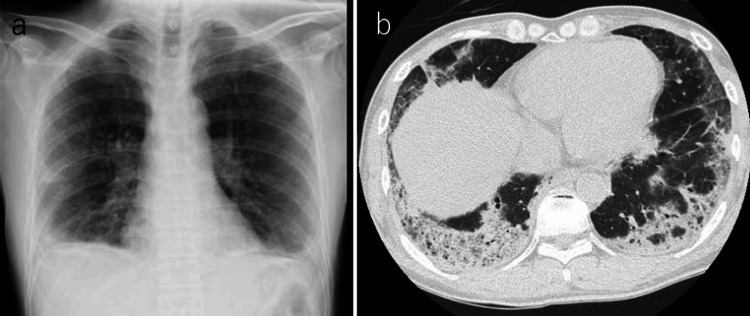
Chest imaging upon admission. a: Chest X-ray revealing a frosted shadow with decreased volume in the bilateral lower lung fields. b: Chest CT image displaying infiltrative shadows and frosted patterns predominantly observed in the outer lower lobes on both sides.
